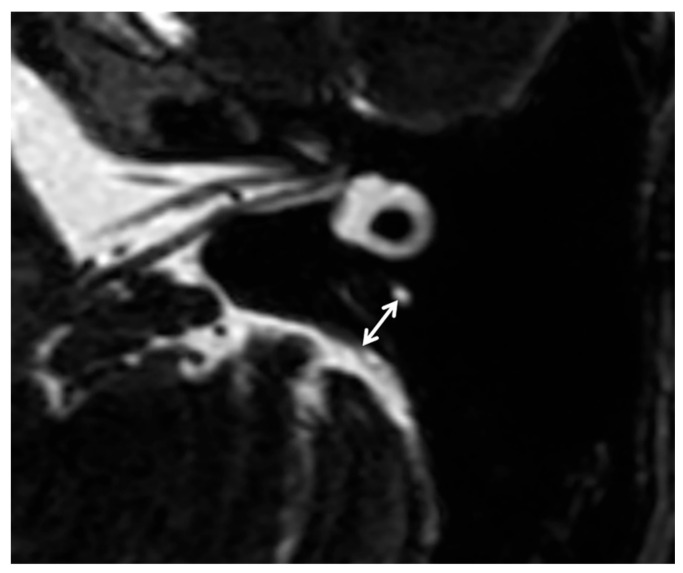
Axial 3D-SPACE MRI scan showing a detailed image of the left ear at the level of the measured distance between the vertical part of the posterior semicircular canal and the posterior fossa.
